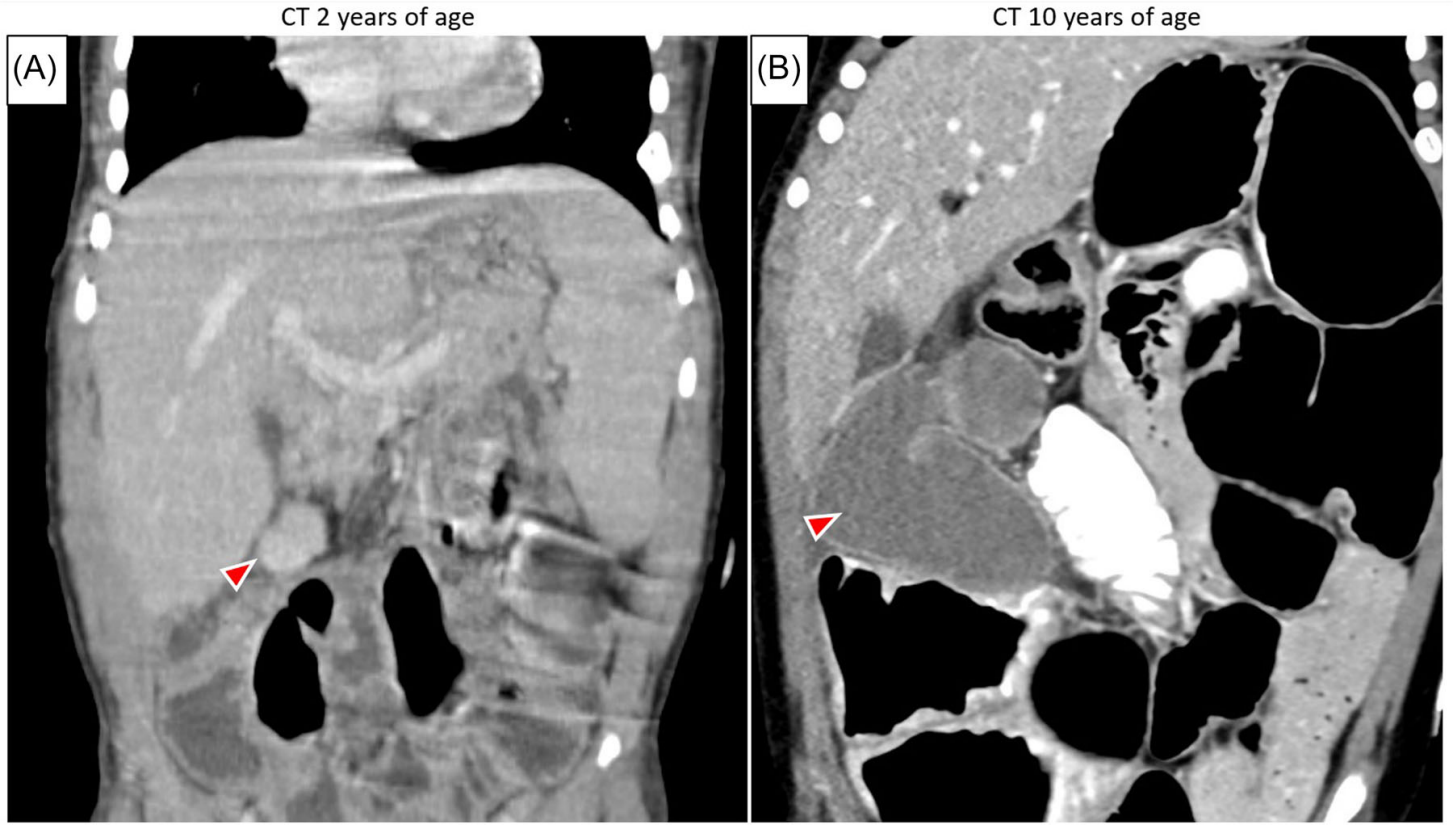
Coronal computed tomography (CT) of the abdomen and pelvis with intravenous (A, B) and oral (B) contrast showing significant growth of the excluded colonic segment over time. Though not detected at the time, the discontinuous segment (arrow) can be visualized in the right upper quadrant of a scan obtained when the patient was 2 years of age (A), 8 years before the events and imaging described in this report (B). Of note, the CT at 2 years of age was obtained during workup for sepsis and revealed an abdominal wall abscess near his gastrostomy tube.
